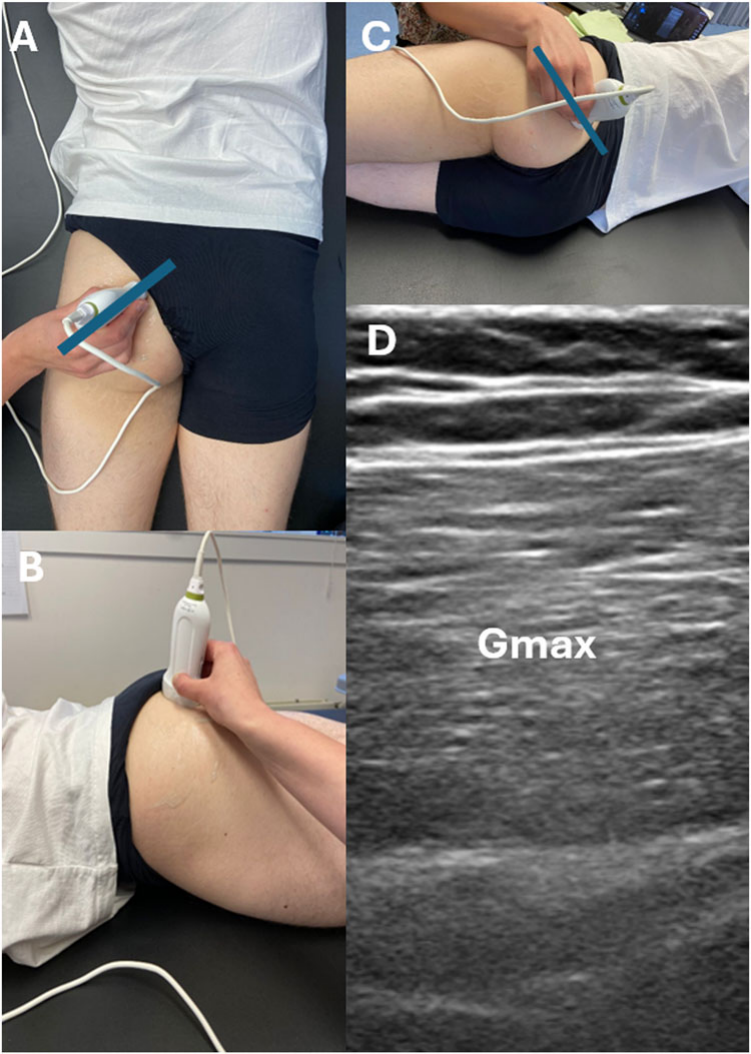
(A) Shows the placement of the probe from the dorsal plane with the reference line in blue, (B) shows the placement of the probe in the transversal plane, (C) shows the placement of the probe after correct positioning the body with the reference line in blue, (D) shows a longitudinal ultrasound image in brightness‐mode of the Gmax (Gmax).
